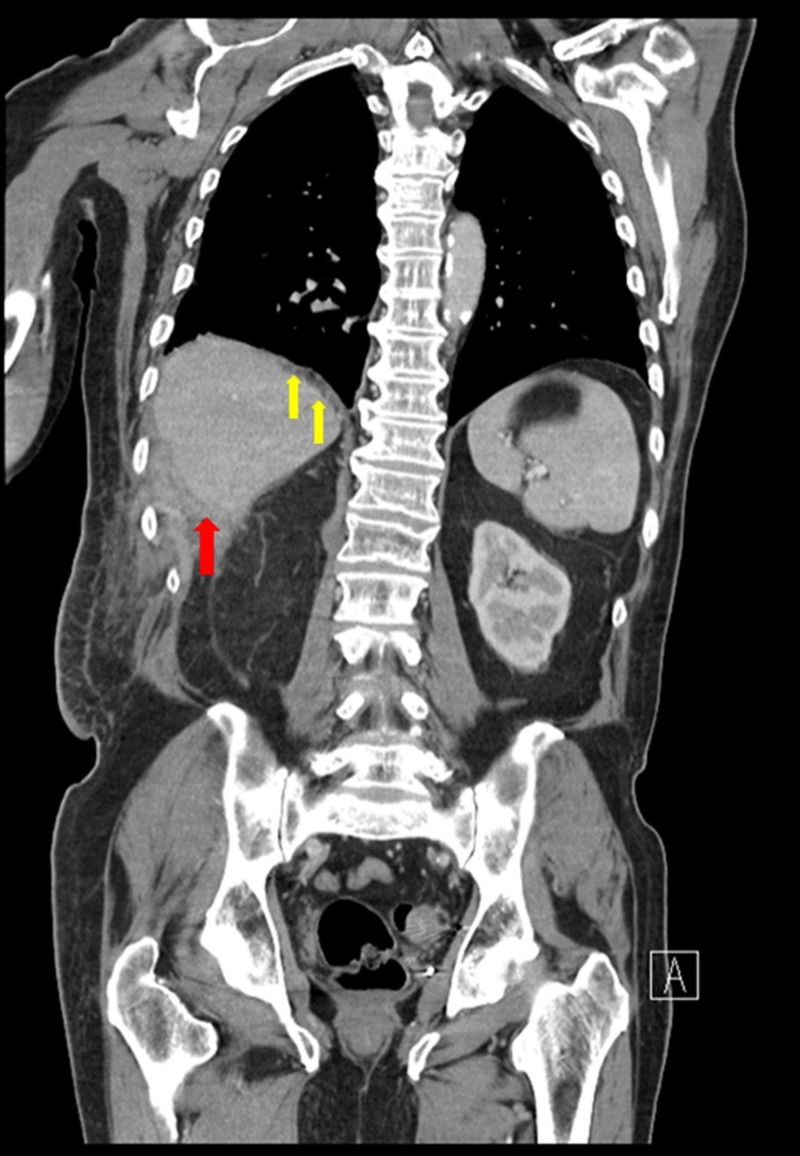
Whole body computed tomography scan showing a diminishing abscess in the inferoposterior aspect of the right hepatic lobe (red arrow) with a diminishing right-sided pleural effusion (yellow arrows).
